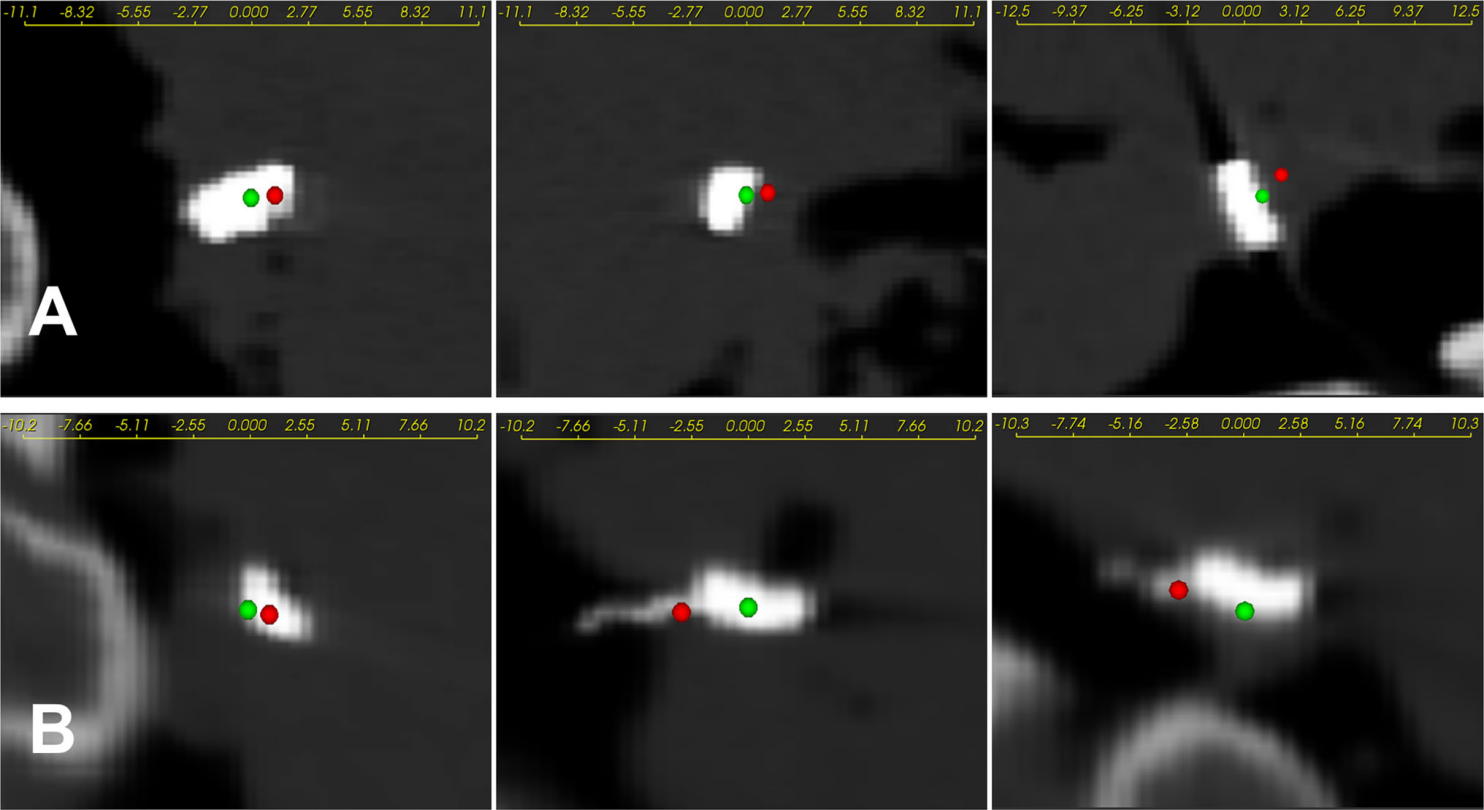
Postoperative CT images of the left (upper) and the right (down) intervention. The cross-sectional planes shown from left to right are sagittal, coronal and axial. Visualization of the actually reached target position as the center on the electrode’s surface closer to the target point (green sphere); and of the target point (red sphere)
